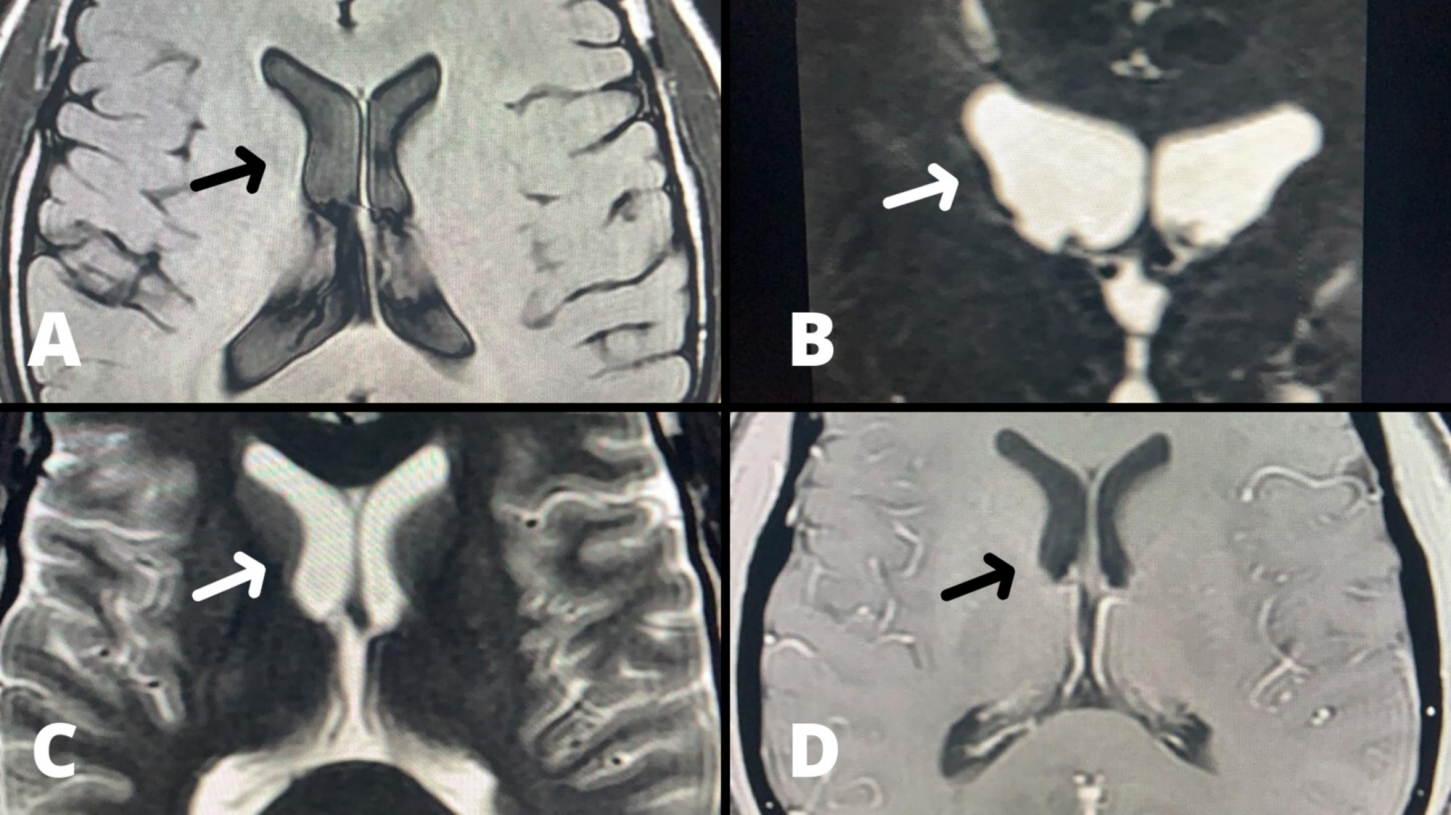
Postoperative axial MRI scan. The images A-D show normalized ventricular size after neuroendoscopic approach.
